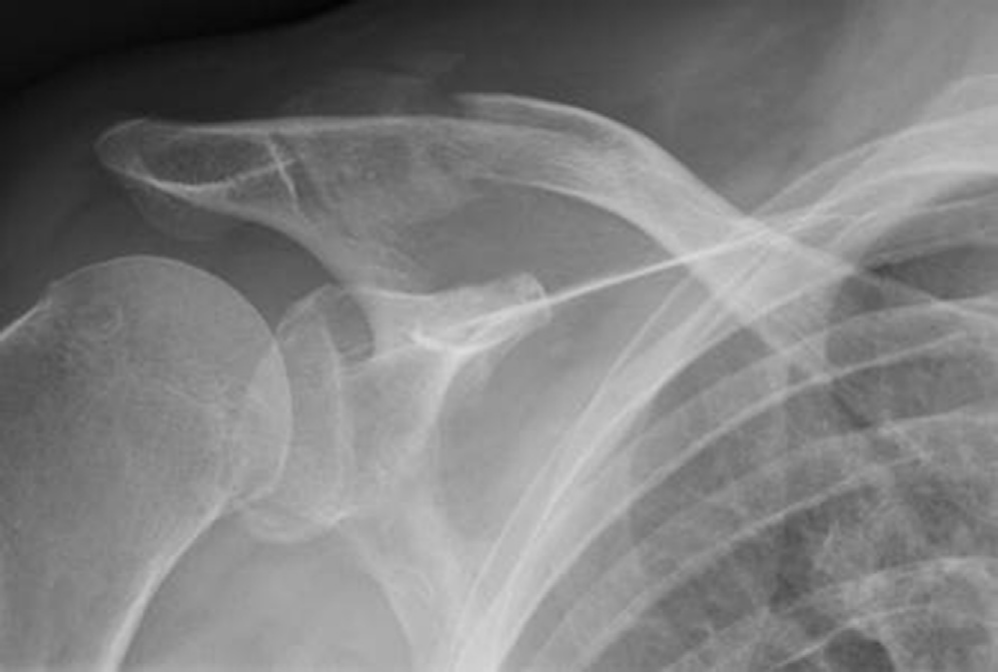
Radiograph of patient with painful nonunion.
